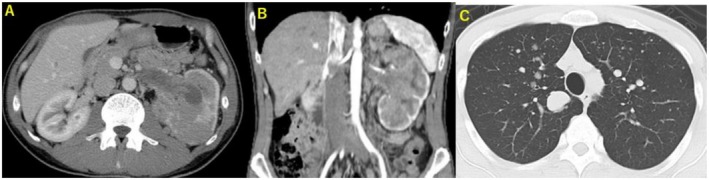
At initial presentation. (A, B) Contrast‐enhanced CT showed a poorly enhancing tumor extending into the left renal vein. (C) Chest CT showed multiple lung metastases. CT, computed tomography.
